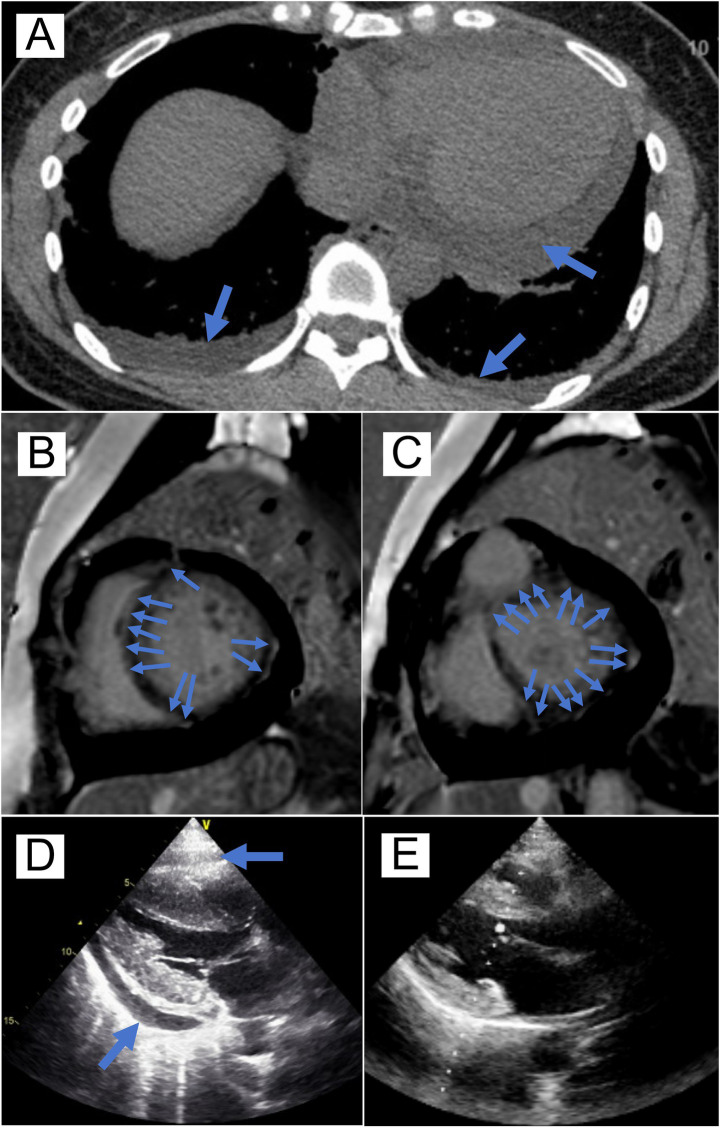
Arrows indicated bilateral pleural and pericardial effusions on chest CT (A). Cardiac MRI with late gadolinium enhancement: arrows indicate patchy non-ischemic subepicardial enhancement in the mid-ventricular segment (B) and basal segment (C) of the left ventricle, consistent with myocarditis. Echocardiography showed left and right ventricular wall thickening. Arrows indicated moderate pericardial effusion (D). A follow-up echocardiogram was performed in October 2024: the LVEF increased to 54%, and no pericardial effusion was found (E).
